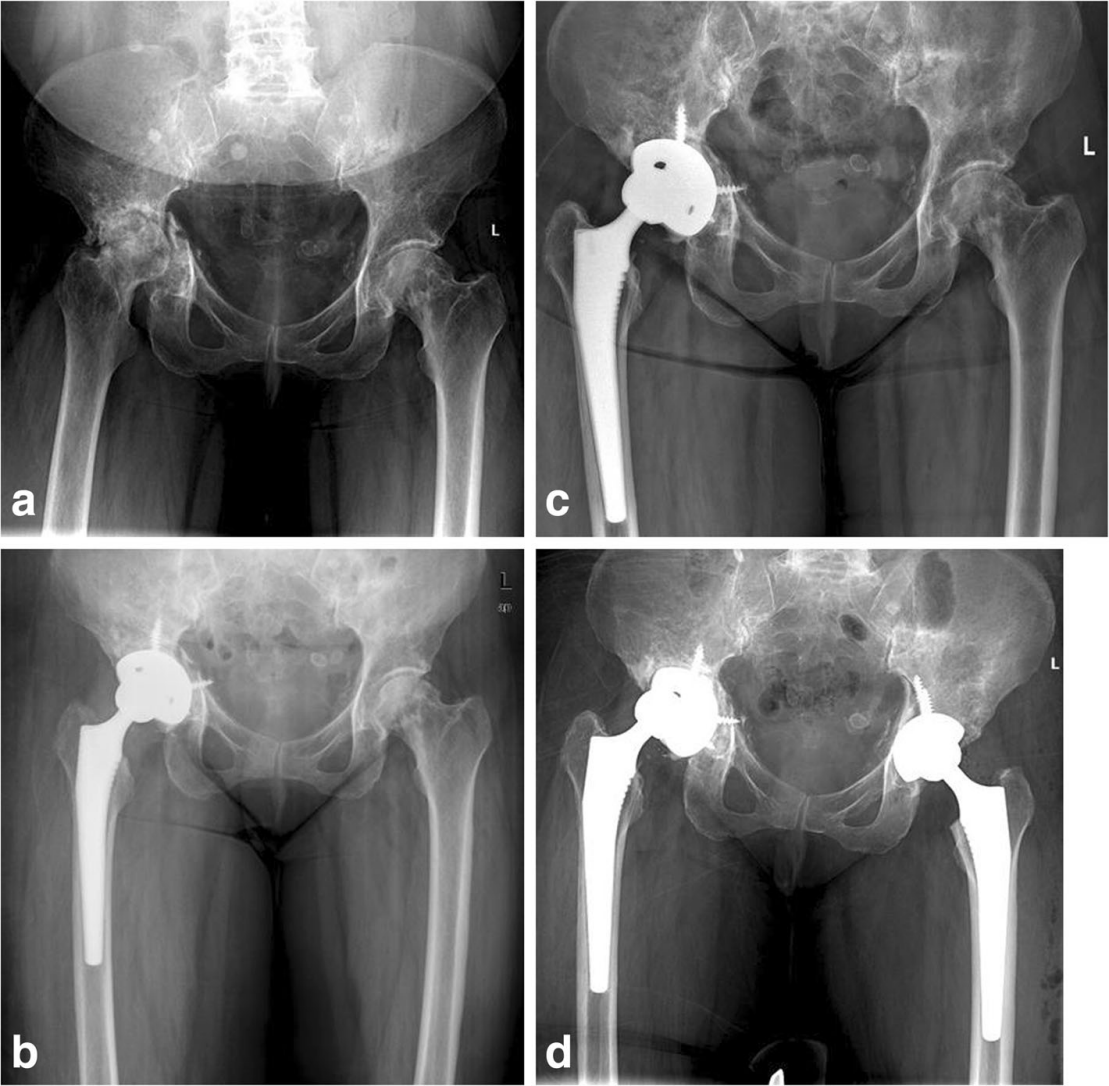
Serial radiographs of a patient with pathological acetabular fracture after pelvic irradiation for vaginal cancer. a Preoperative X-ray. b Immediate X-ray after surgery. c Migration of the cup observed at 1.5 years after surgery. d No intervention of the loosened cup due to no symptom and total hip arthroplasty of contralateral hip
